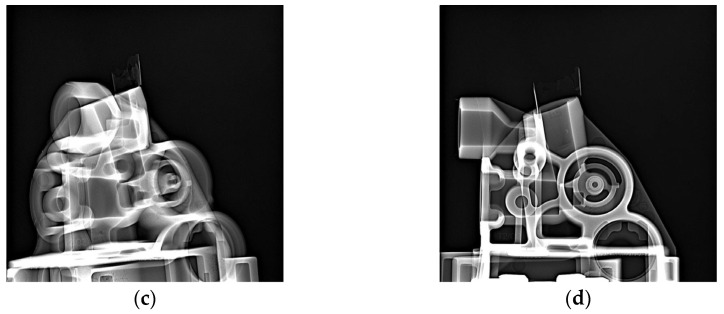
X-ray inspection for maximum (about 35 mm) value of biscuit thickness in specific sockets: socket C1: (a) area A—rate value 3, (b) area B—rate value 2; socket C2: (c) area A—rate value 3, (d) area B—rate value 2.
